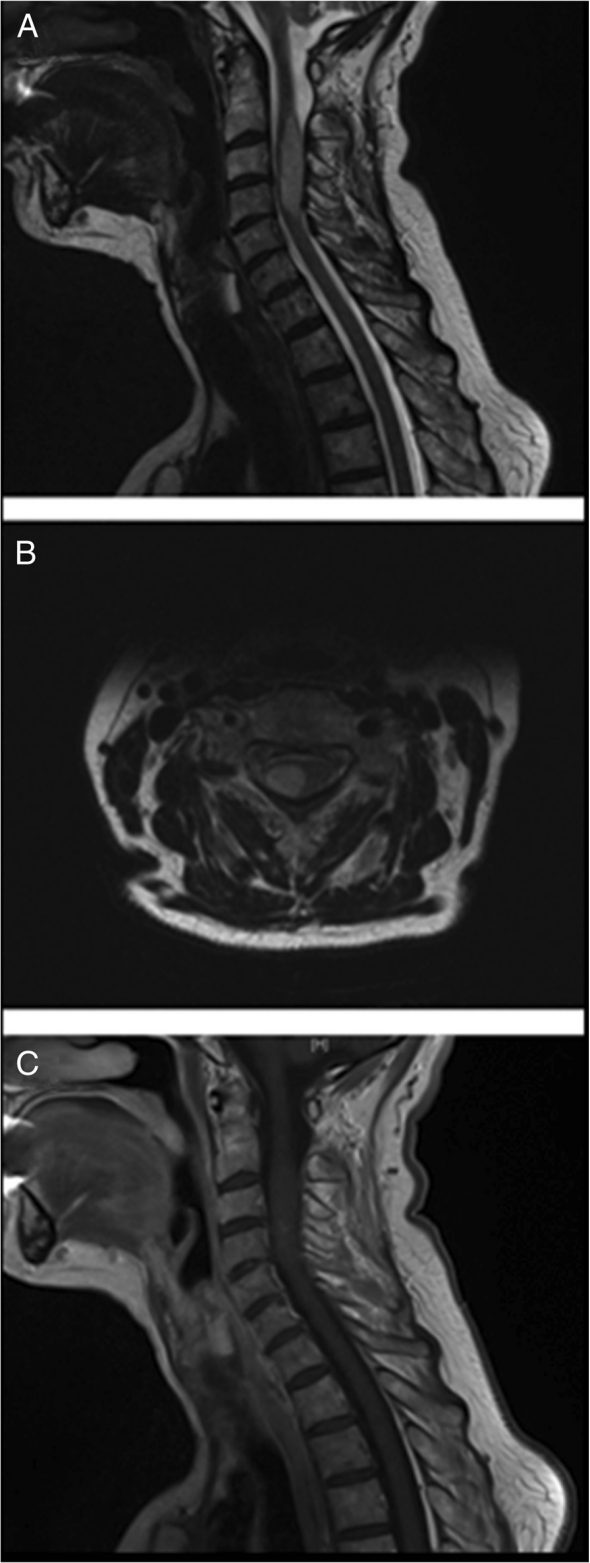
MRI of cervical spine: Sagittal and axial T2-weighted MRI images show an intramedullary tumor (A and B). T1-weighted images with contrast enhancement show a slight contrast uptake (C).
